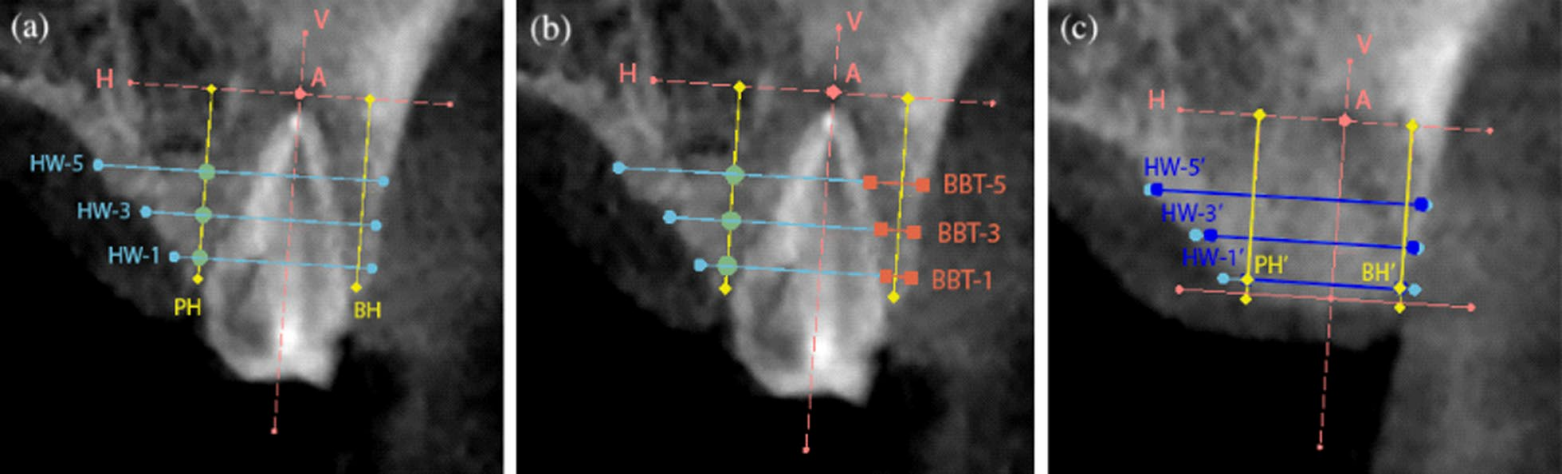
Linear measurements on CBCT images of the case treated following the alveolar ridge preservation protocol. a) Preoperatively the horizontal analysis was performed at 1, 3 and 5 mm (HW-1, HW-3, HW-5) and the vertical analysis was performed on the buccal and palatal side (BH, PH); b) preoperatively the buccal bone thickness was measured at 1, 3 and 5 mm (BBT-1, BBT-3, BBT-5); c) postoperatively the horizontal analysis was performed at 1, 3 and 5 mm (HW-1, HW-3, HW-5) and the vertical analysis was performed on the buccal and palatal side (BH, PH)
